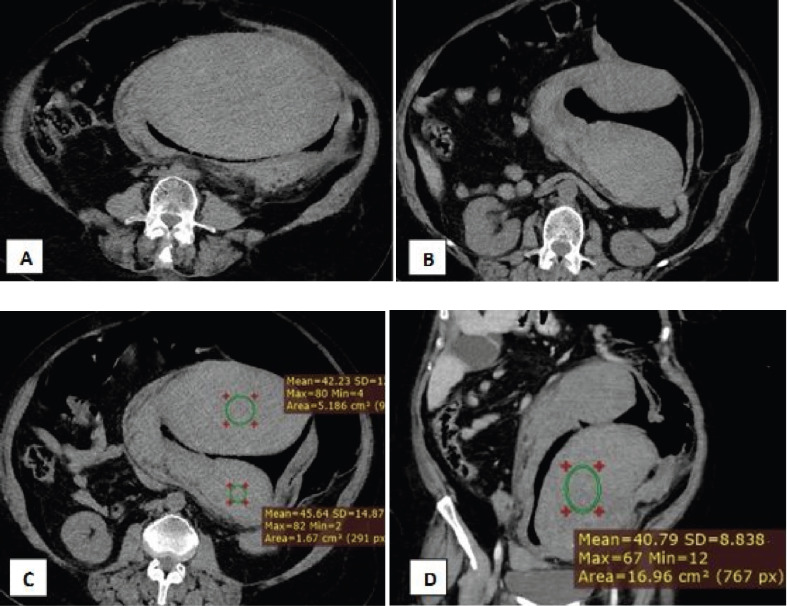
Axial [A-C] and coronal [D] Computed Tomography of abdomen showing homogeneously hyperattenuating submucosal mass involving the sigmoid colon [attenuation 40-45 Hu] which extends along the entire length of the sigmoid colon suggested a hematoma, led to the decision for surgical intervention
